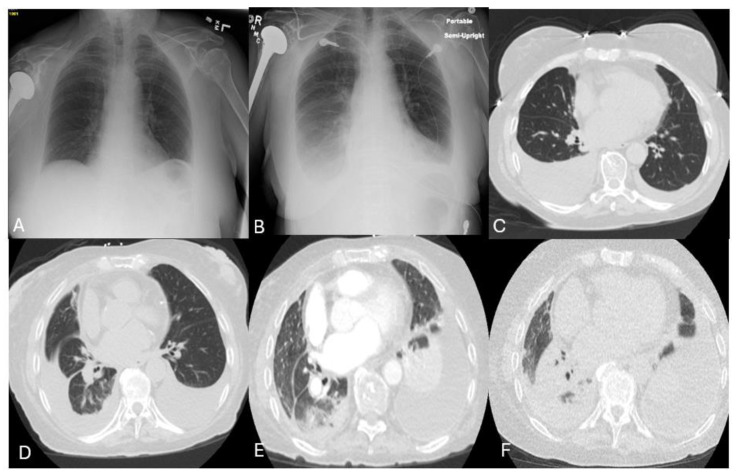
Radiological imaging of the index patient across various time frames. Radiological imaging of the index patient. (A) Baseline Chest X-ray before TKI initiation which is normal. (B) Chest X-ray performed in January 2018 while being on Dasatinib maintenance. (C) CAT scan performed in January 2018 while on Dasatinib, which shows bilateral pleural effusion (right-sided more than left-sided). (D) CAT scan performed while on Nilotinib therapy; this time pleural effusion is dominant on the left sided, while the right side is reduced. (E) CAT scan in 2022 while on Bosutinib. (F) CAT scan performed during index admission showing worsening left-sided pleural effusion with underlying lung collapse and right-sided pleural effusion with underlying collapse/consolidation in the form of air bronchograms.
